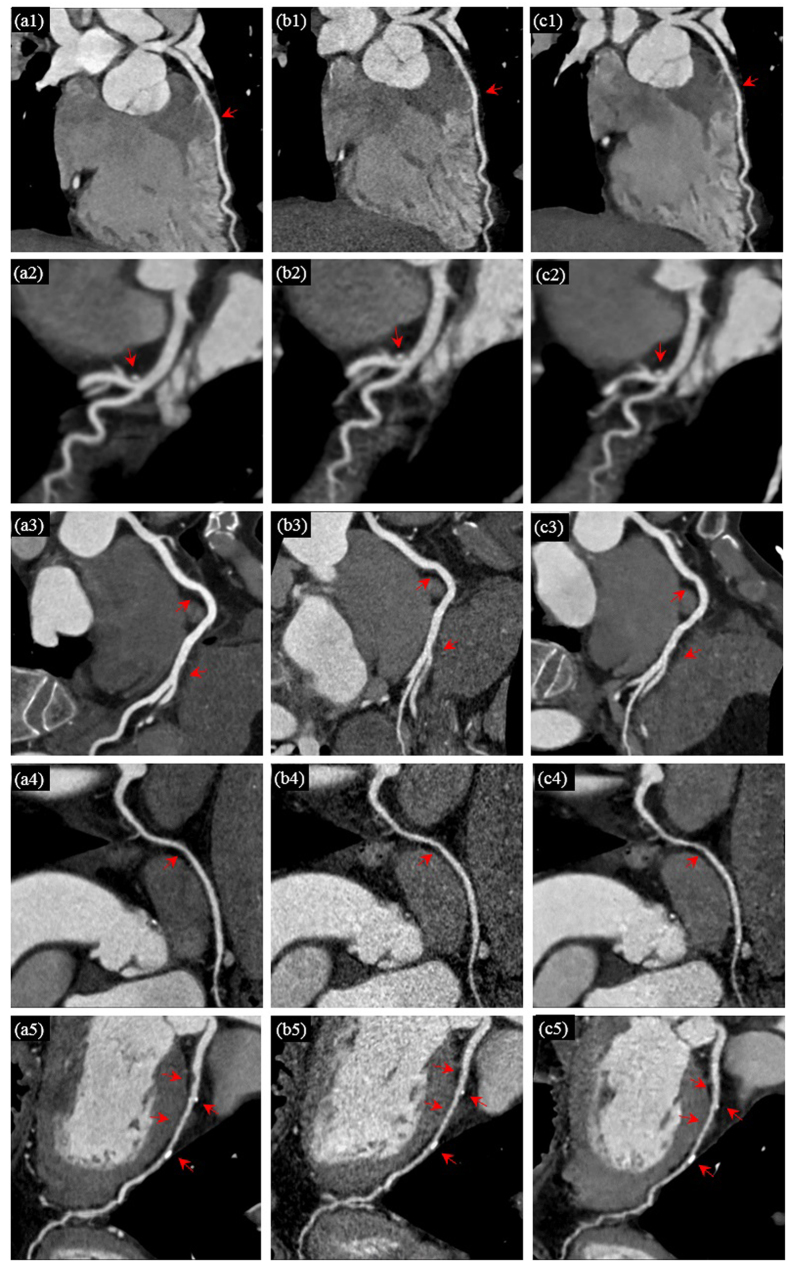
Curved planar reformatted CT images of five patients with vascular abnormalities. The first, second and third columns correspond to the CPR images derived from reference SDCT data, the LDCT data and the 3D SR processed LDCT data. The first row shows left anterior descending artery (LAD) with coronary myocardial bridge and mild stenosis (arrows); the second row shows anterior descending with hybrid plague and moderate stenosis (arrows); the third and fourth row show right coronary artery (RCA) with non-calcified coronary plaque and mild stenosis (arrows); the fifth row shows left anterior descending artery (LAD) with multiple calcified and non-calcified coronary plague, mild to moderate stenosis and coronary myocardial bridge (arrows).
